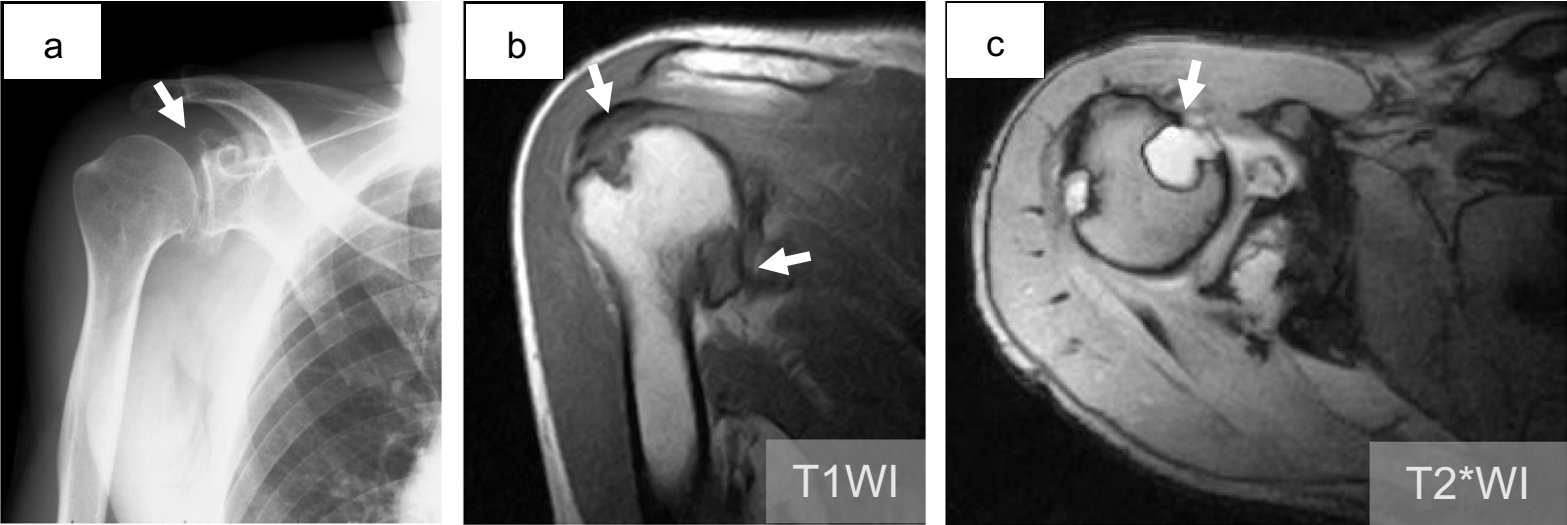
Radiography and MRI taken 6 years prior to diagnosis. a Radiography reveals a expanding osteolytic lesion in the glenoid of the scapula (arrow). b, c MRI showed intra-articular masses with iso-intensity on T1WI and high-intensity on T2*WI. These masses caused erosion of the humeral head (arrows)
